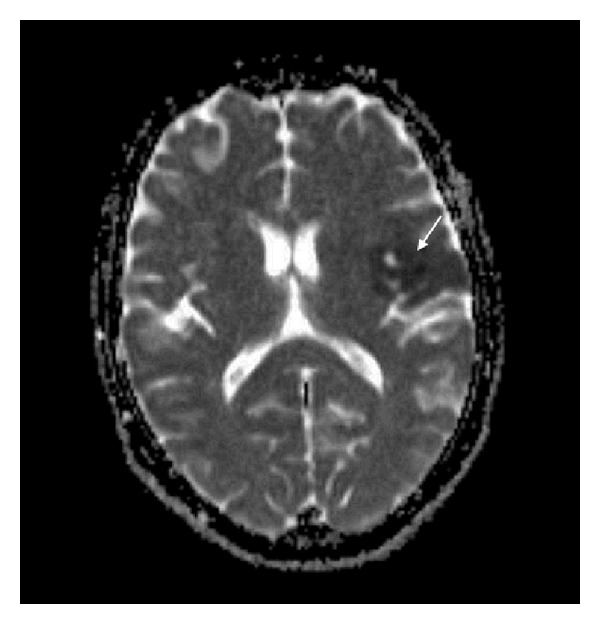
Axial ADC-MRI scan demonstrating low intensity lesion at the left frontal region consistent with acute infarction.
